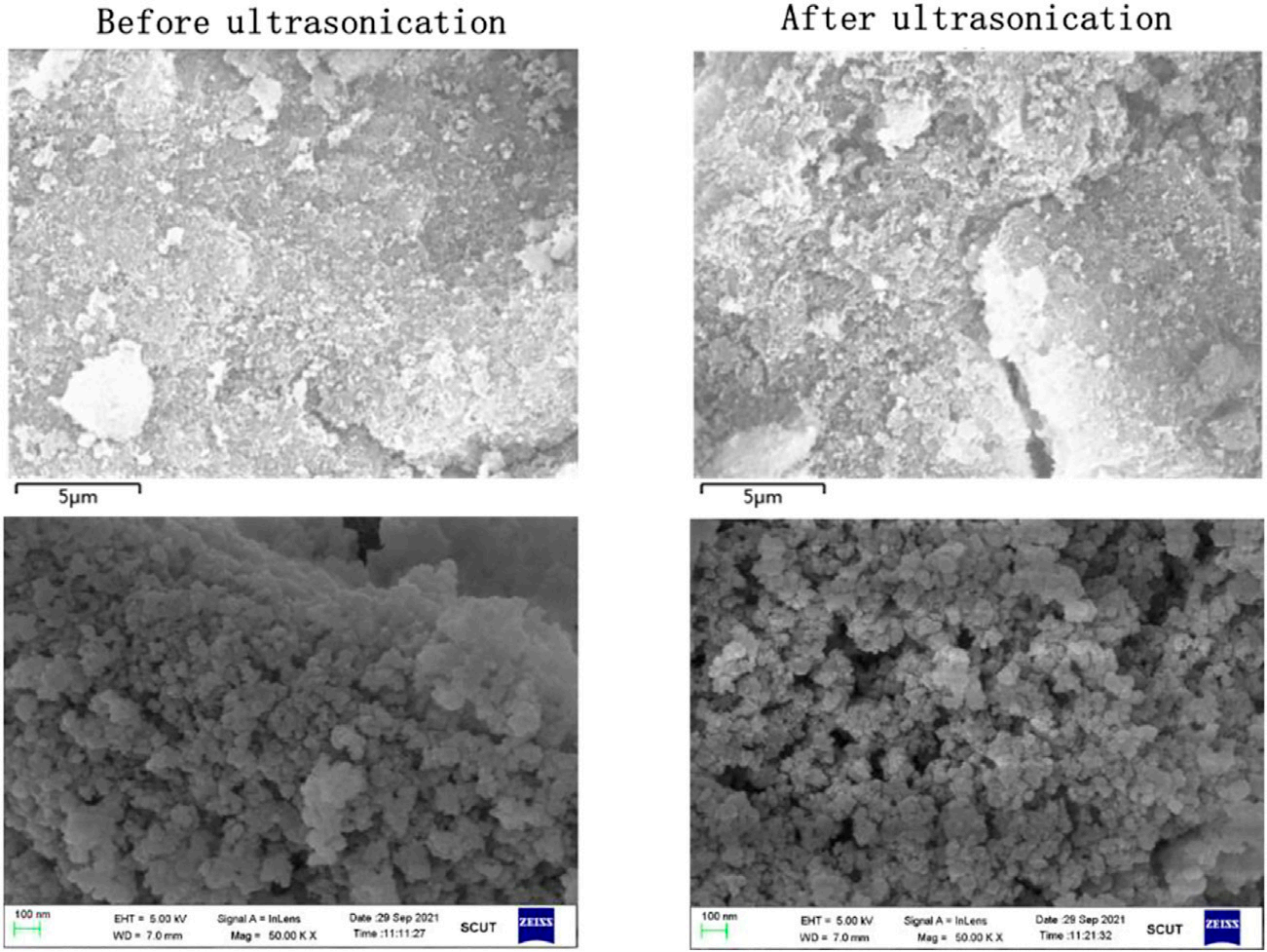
Topography change of chromium sludge (EPS1) before and after ultrasonic treatment.
